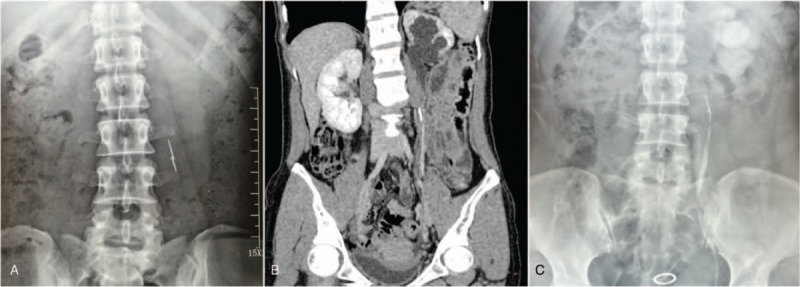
(A) KUB showed a foreign body on the left side of the spine early in 2014. (B) Computed tomography (CT) scan revealed moderate hydronephrosis of the left kidney. (C) Retrograde pyelogram showed stricture of the upper ureter in the region near the foreign body.
